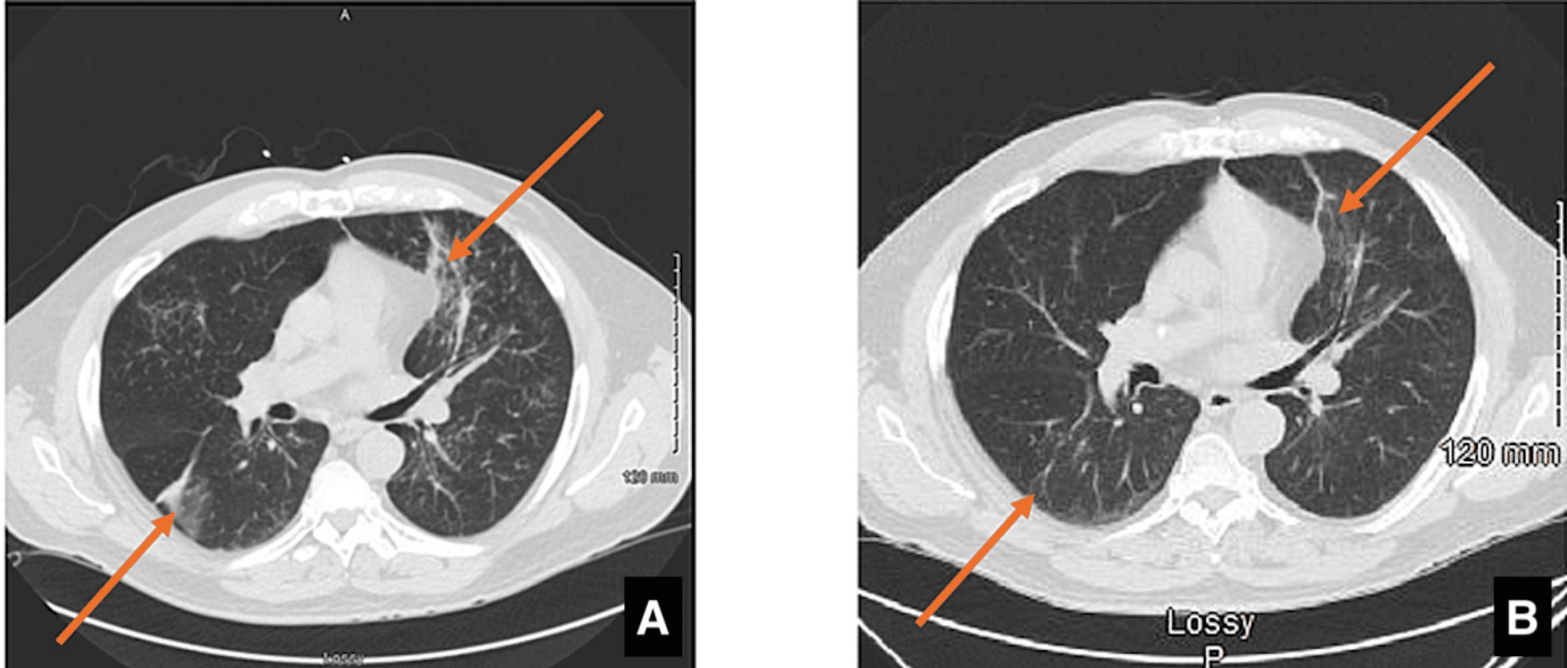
Lung CT scan (A) CT scan with perihilar bronchial wall thickening, reactive nodes, and diffuse ground-glass peripheral opacities; (B) CT scan after antibiotic therapy
